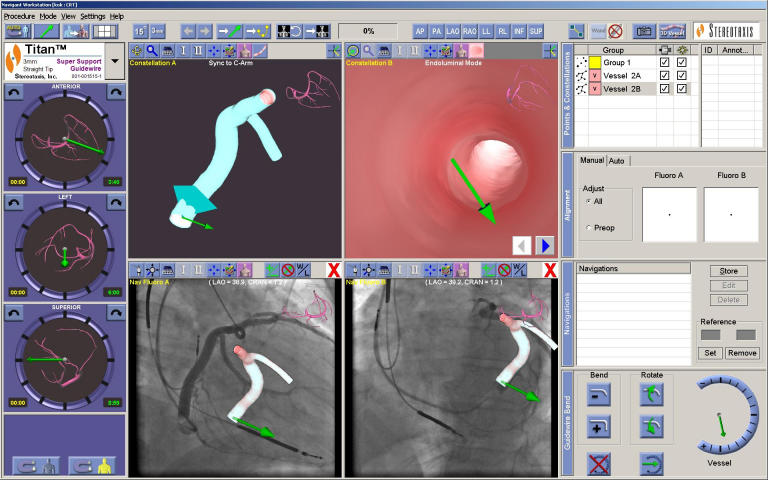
PAIEON - This image again shows the Navigant screen with the reconstruction overlaid on the radiographic images saved during the angiogram. The image above the LAO radiographic image shows the 3D reconstruction and the image above the RAO shows a fly-through image from the reconstruction. This is then used to navigate the magnetically enabled guidewire into the CS side branch. The other features which can be seen are aids to navigation.
